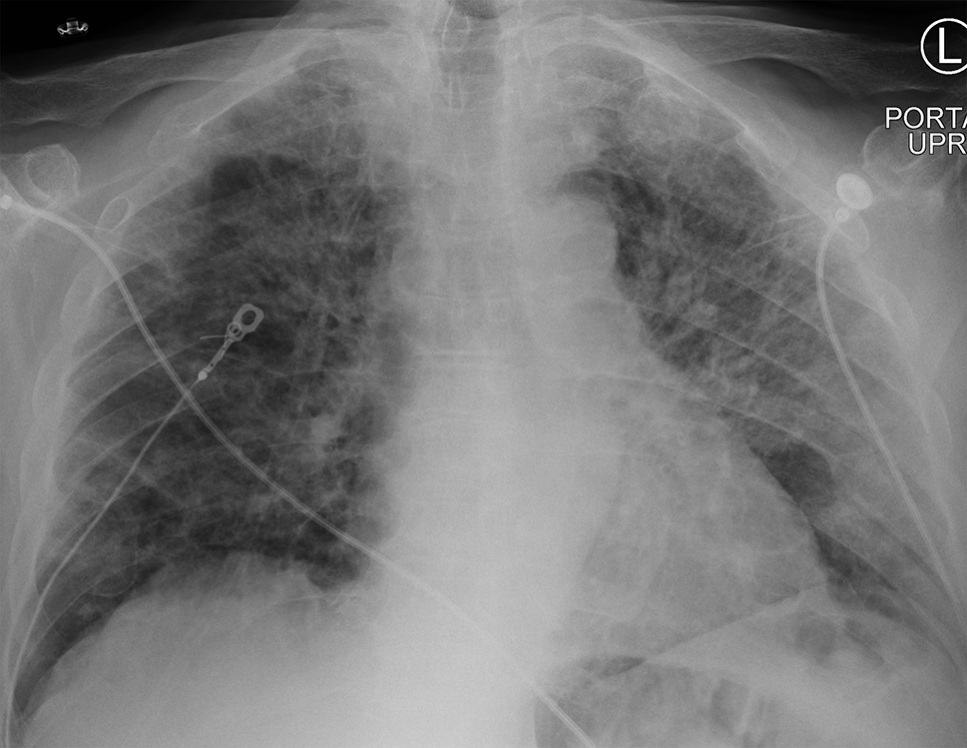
Interval Chest X-Ray approximately 10 h after admission, re-demonstrating bilateral but with significantly worsening left sided pulmonary opacities
